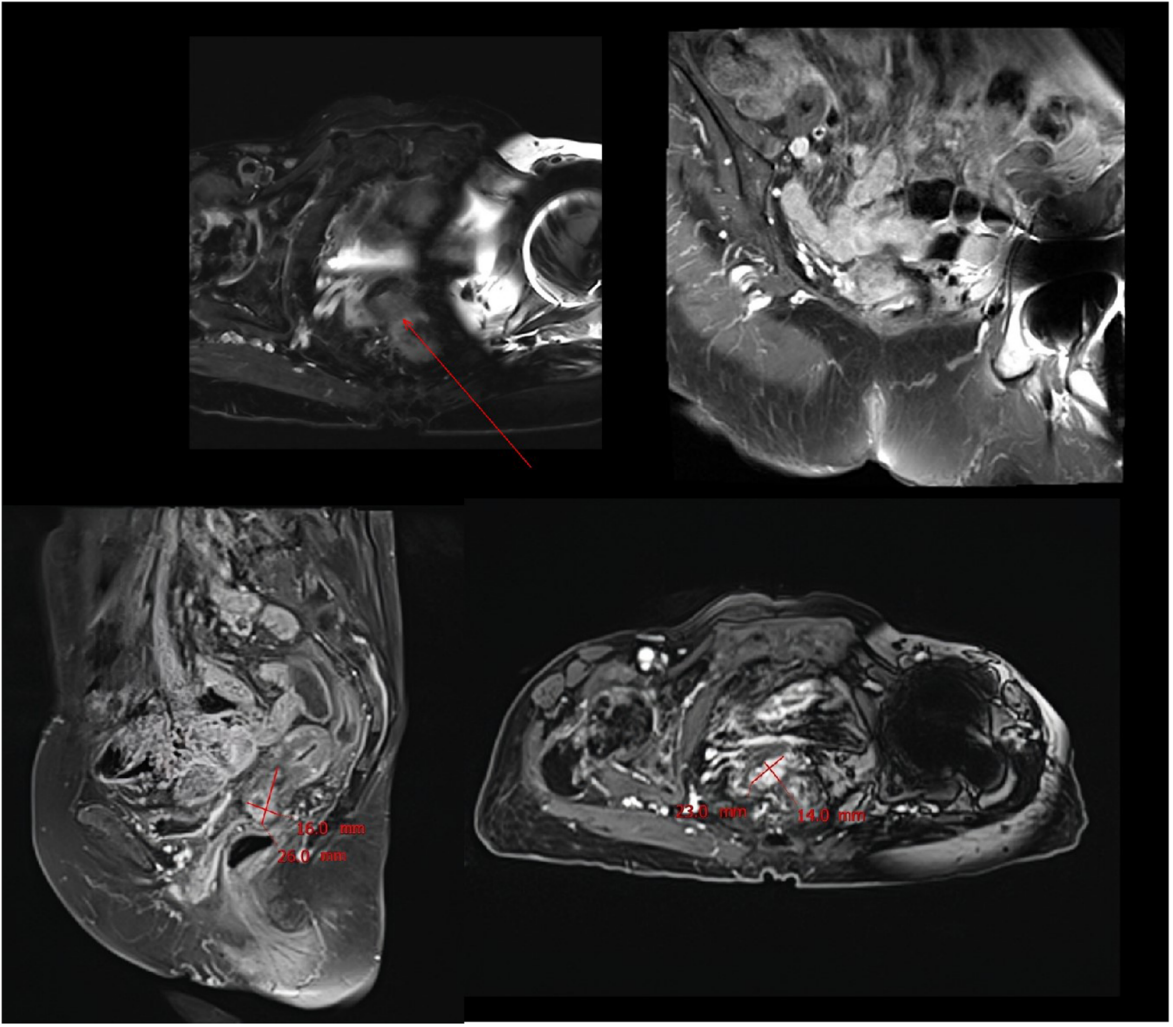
MRI scan performed after 13 months of combination therapy showed significant tumor recurrence.
